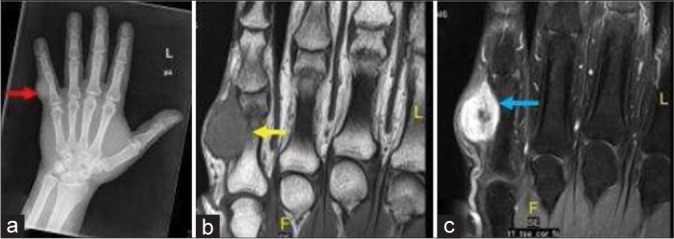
A 20-year-old male with nodular fasciitis of finger who presented with painful rapidly developing swelling in the proximal phalynx of ring finger. (a) Plain radiograph of the left hand demonstrates a well-defined soft tissue swelling in the proximal phalanx of the little finger (red arrow). Note absence of bony destruction or scalloping. (b) Coronal noncontrast T1-weighted image shows that the lesion is well defined and isointense to the muscle (yellow arrow). (c) The lesion demonstrates intense enhancement on fat saturated post-contrast T1-weighted image and is difficult to differentiate from sarcoma or giant cell tumor of tendon sheath.
